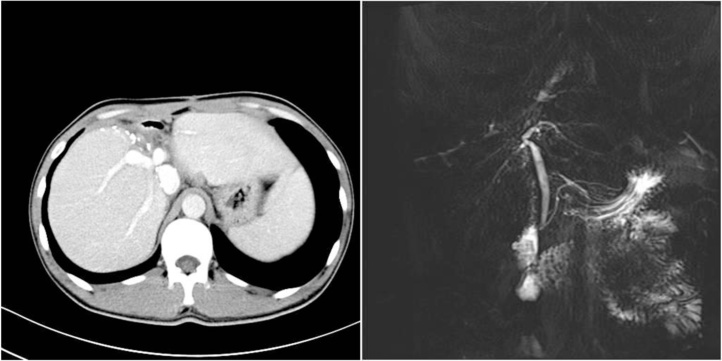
a: CT scan (6 months after operation); b: MRCP (6 months after operation).
